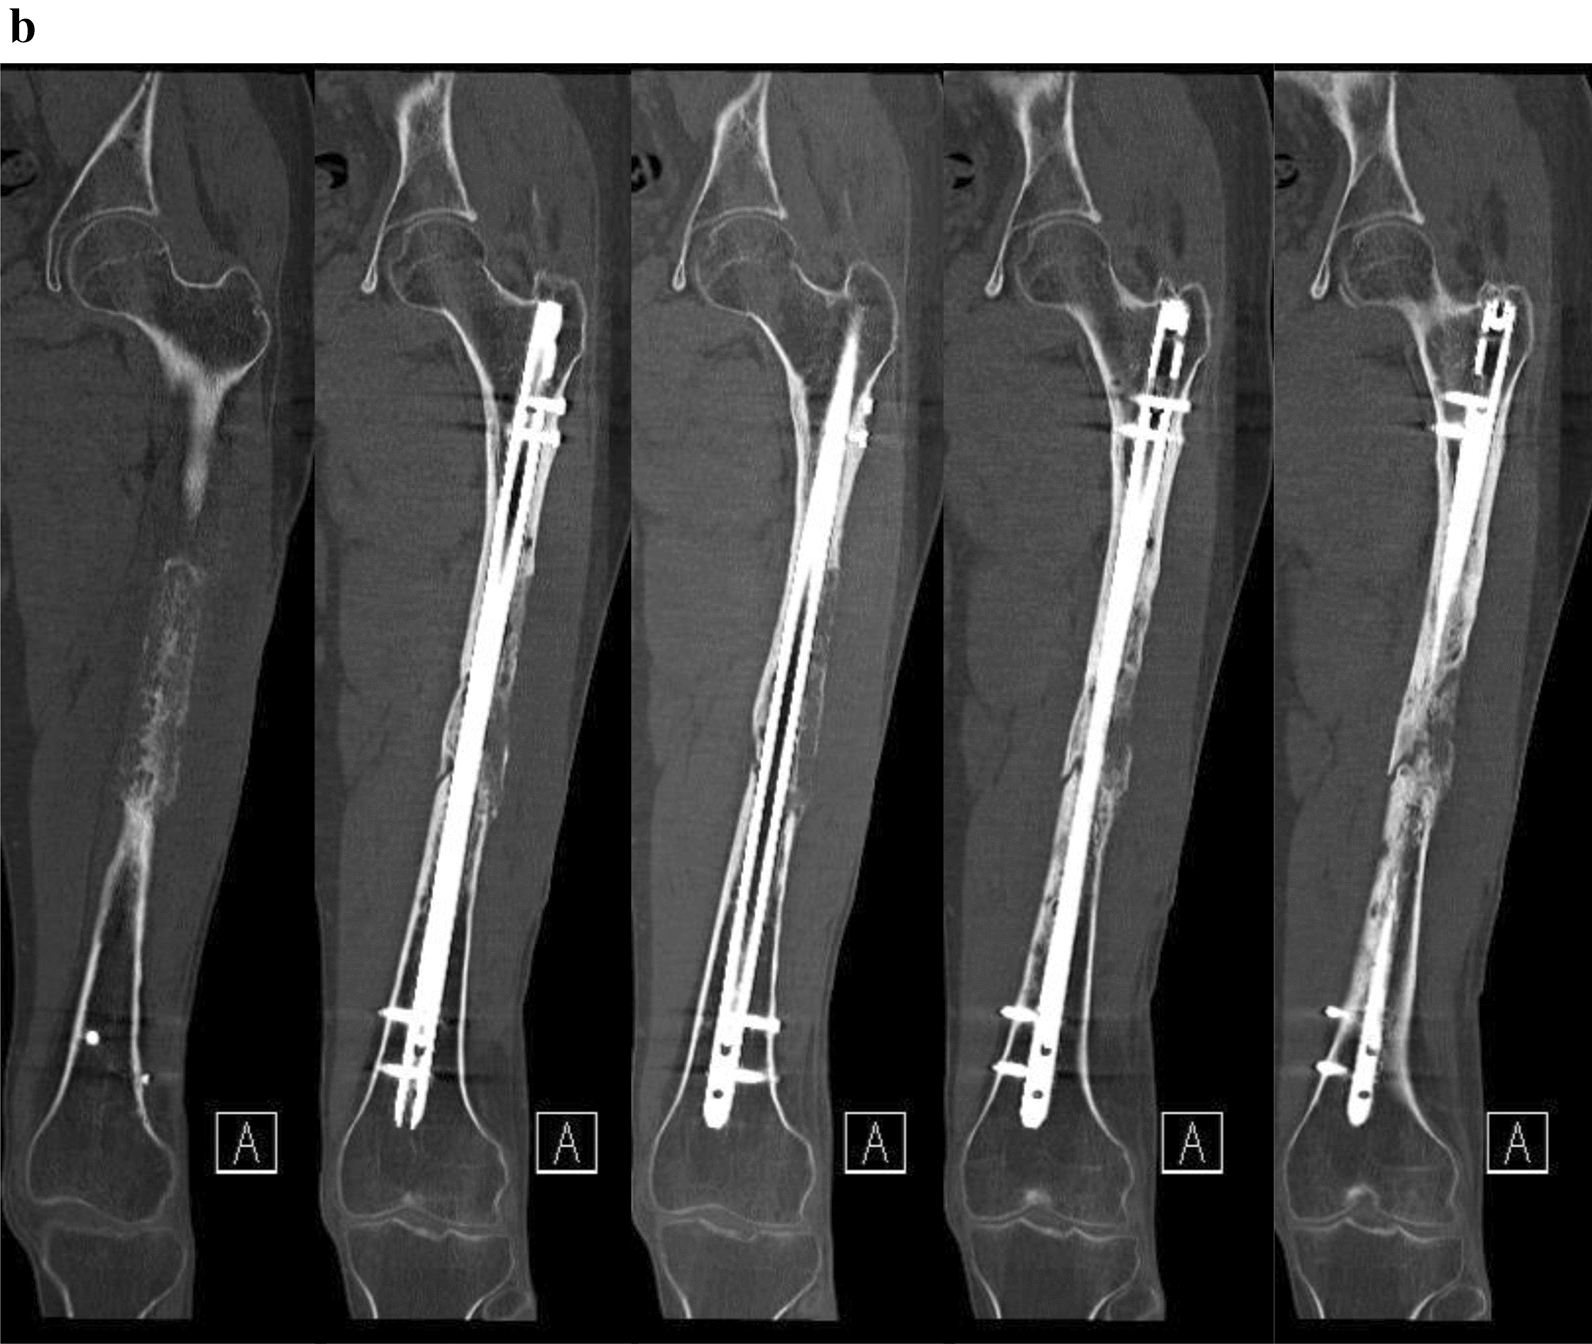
a X-ray 12 months after surgery shows delicate but adequate bone formation. b CT-scan 12 months after surgery confirms almost complete bony fusion of the critical-sized defect with partial degradation of the mPCL–TCP scaffold
